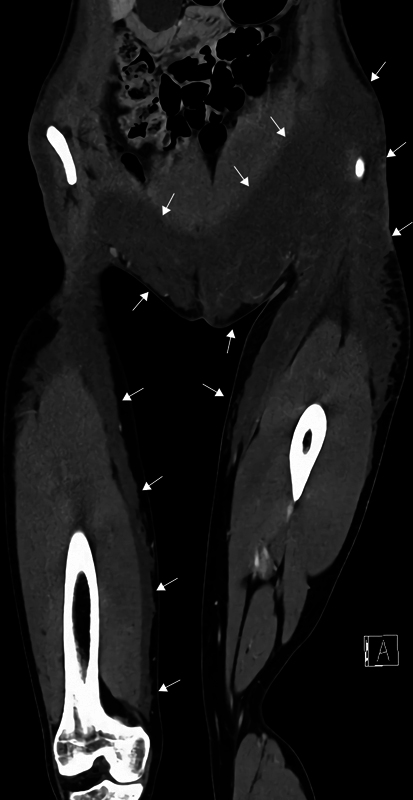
Contrast-enhanced computed tomography (CT) scan (coronal view on the level of spina iliaca anterior superior) showing migration of the filler, highlighted by arrows, extending from the hips to the lower abdomen, into both inguinal regions and the left labia majora, and down to the anteromedial part of the thighs. On the right side, the filler extends down to the level of the knee.
